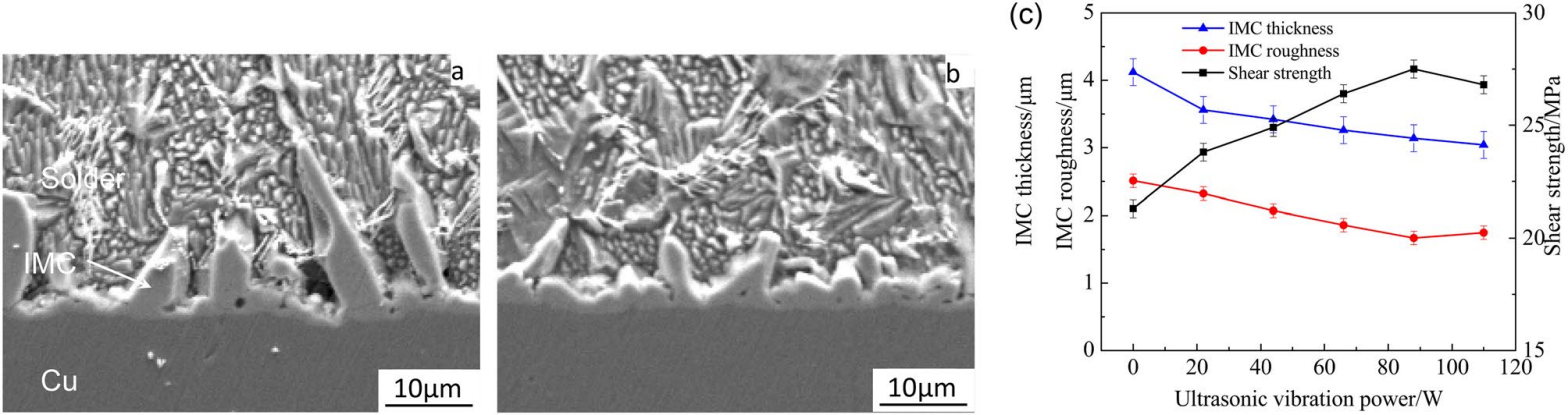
Effects of ultrasonic vibration power on ultrasonic-assisted Sn2.5Ag0.7Cu0.1RE0.05Ni/Cu solder joints: (a) 0 W, (b) 88 W, (c) IMC thickness, IMC roughness and shear strength of the solder joints.
